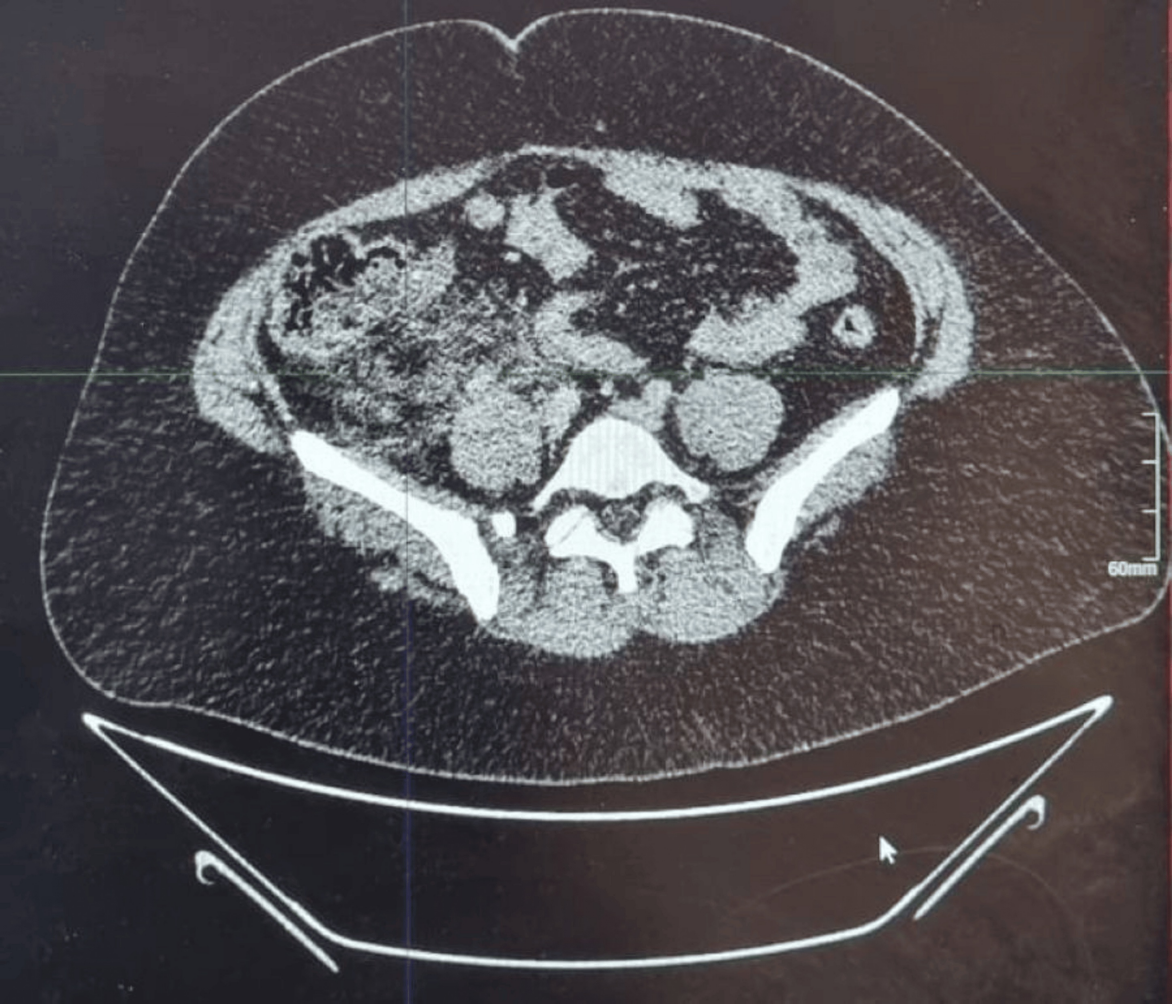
Another preoperative contrast-enhanced CT of the abdomen and pelvis revealed thickening of the adjacent cecal wall and terminal ileum, suggesting a localized inflammatory process A periappendiceal gas locule and a small amount of adjacent free fluid were also observed CT: computed tomography
